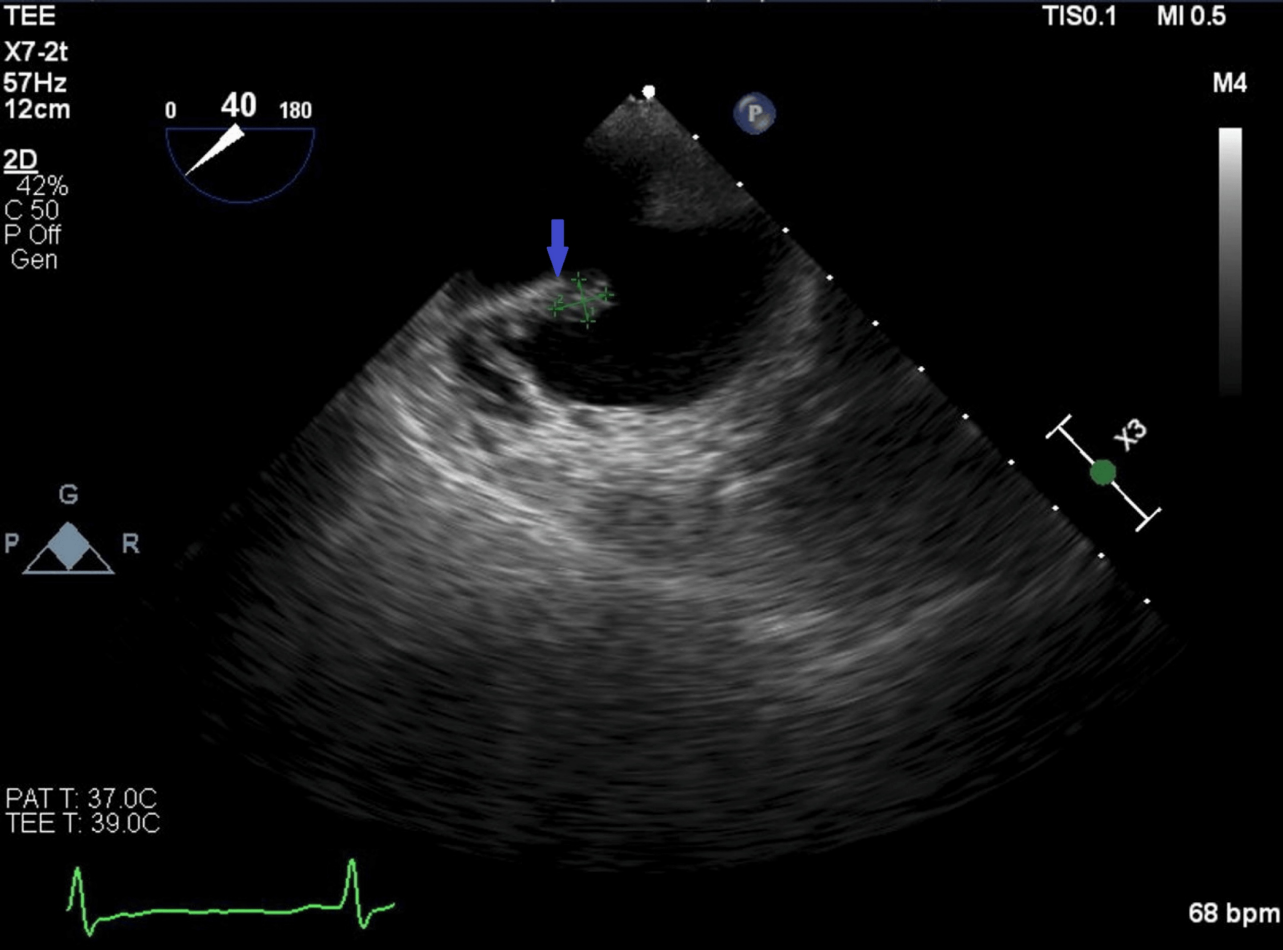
Transesophageal echocardiogram showing the eustachian valve (blue arrow) and mass corresponding to suspected vegetation on the eustachian valve (green highlight).
